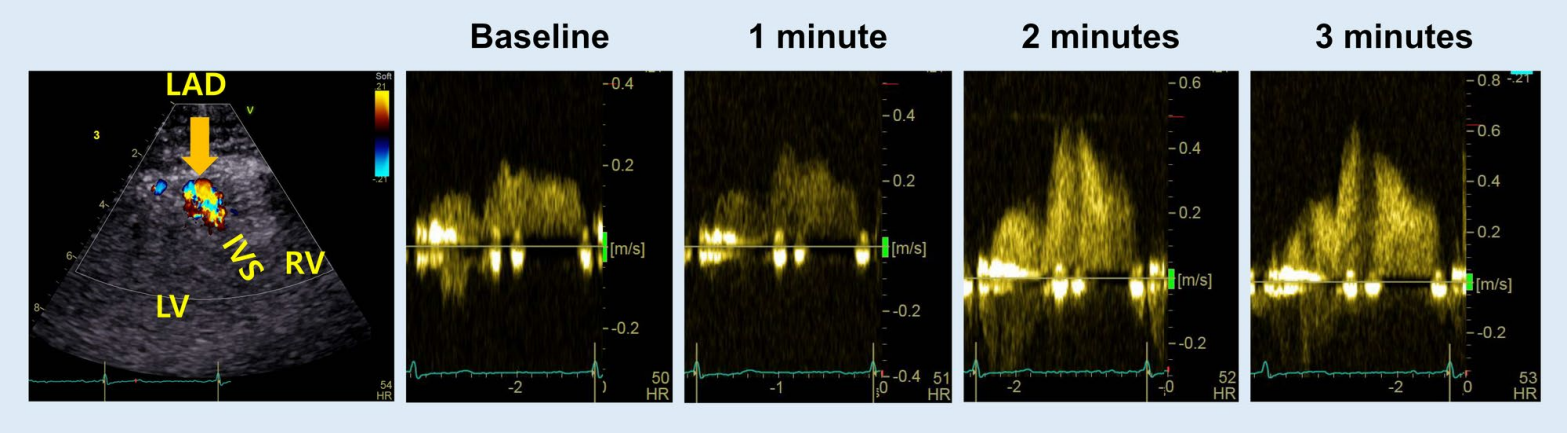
Transthoracic echocardiographic assessments of CBF velocities. The color Doppler flow of the distal left anterior descending artery was examined from the modified apical four-chamber view of the anterior interventricular groove. Pulsed-wave Doppler images were acquired at baseline, and 1, 2, and 3 min after adenosine infusion in the same part of the artery. *CBF* coronary blood flow, *IVS* interventricular septum, *LAD* left anterior descending artery, *LV* left ventricle, *RV* right ventricle
